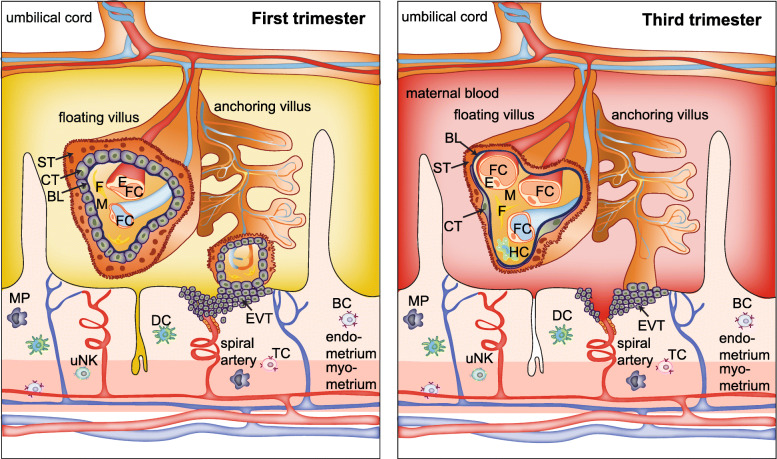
Scheme of the human placental barrier in early and late pregnancy. In the first trimester, the placental barrier consists of the syncytiotrophoblast (ST), cytotrophoblasts (CT), basal lamina (BL) and the endothelial cells (E) of the fetal capillaries (FC). Other cell types in the villous mesoderm include fibroblasts (F) and Hofbauer cells (HC). Various immune cells are also present in the maternal decidual tissue, including dendritic cells (DC), macrophages (MP), uterine natural killer cells (uNK), T cells (TC) and B cells (BC). Extravillous trophoblasts (EVT) of the anchoring villi invade the maternal spiral arteries (SA) and form a plug that prevents entry of maternal blood into the intervillous space, and uterine glands (UG) provide histiotrophic nutrition. After the first trimester, the EVT plug is released and placental villi are now surrounded by maternal blood. Towards the end of pregnancy, the placental barrier decreases in size by thinning of the ST layer and spreading of the CT layer, and the FCs move towards the periphery of the floating villi
